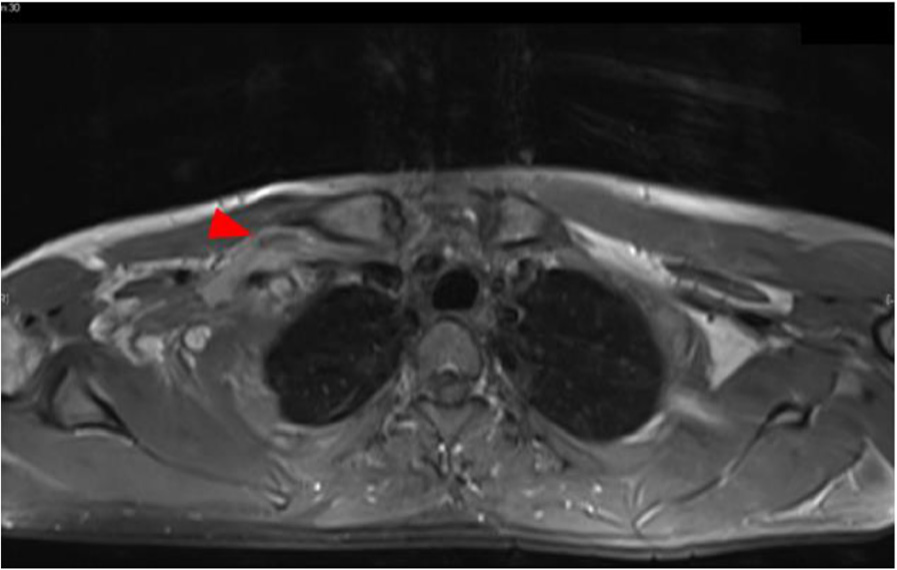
MRI of infraclavicular level. Abnormal exposure of the right brachial plexus, indicating a partial injury.
